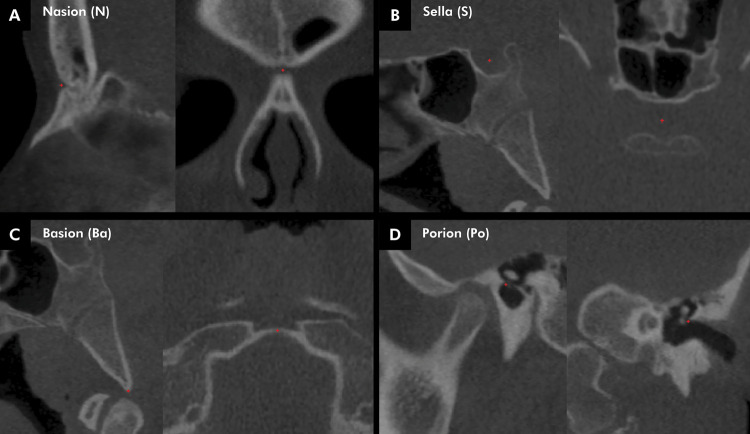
Landmarks in red color applied to multiplanar CBCT slices. Nasion (A), sella (B), basion (C) and porion (D) cephalometric points.
